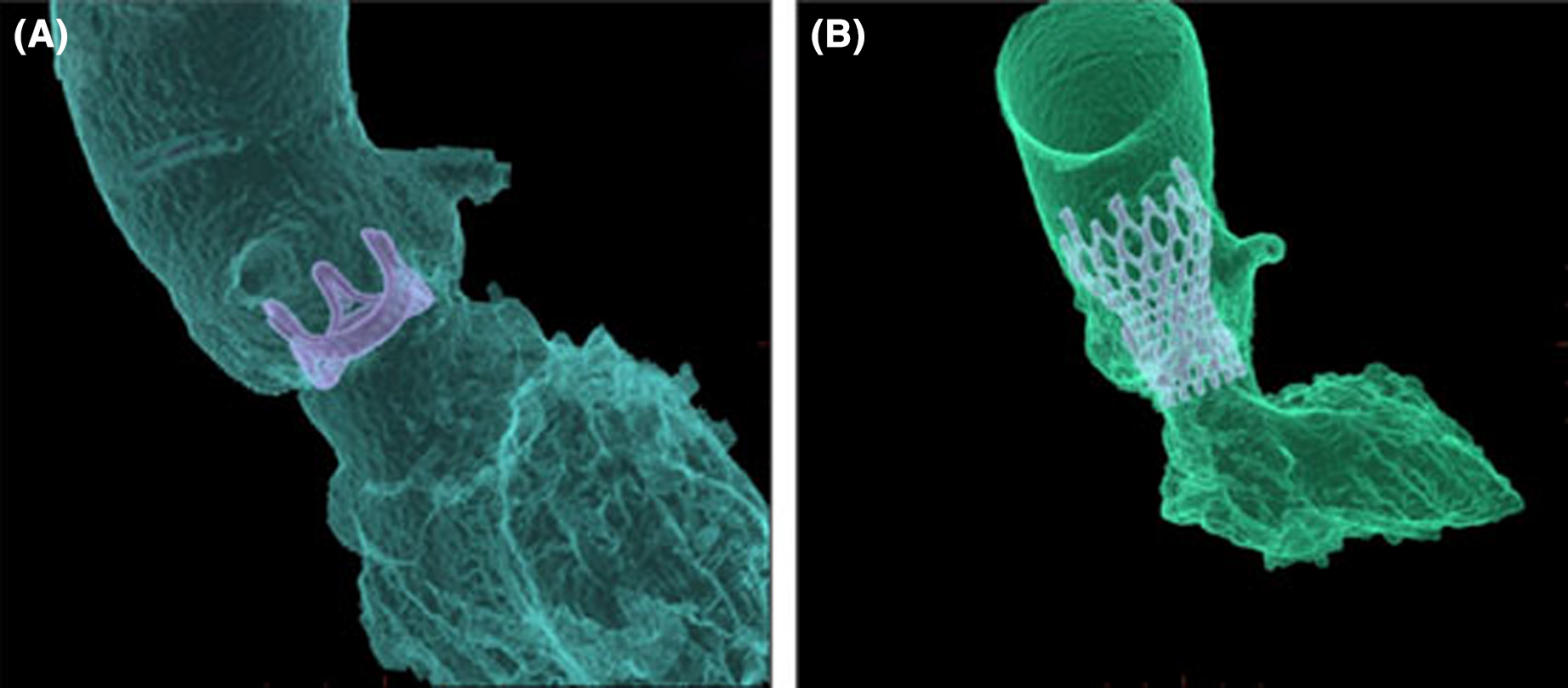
CT three‐dimensional reconstruction of aortic root (A) Preoperation; (B) postoperation.
